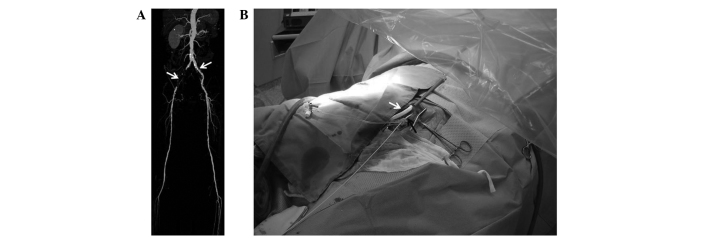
Iliac stenting and crossover femorofemoral bypass. (A) Preoperative CTA showing long segment occlusion of the right iliac artery and focal stenosis of the left common iliac arteries. (B) Image during hybrid surgery showing the artificial graft for a bypass and the vascular introducer sheath for endovascular procedure. CTA, computed tomography-angiography.
